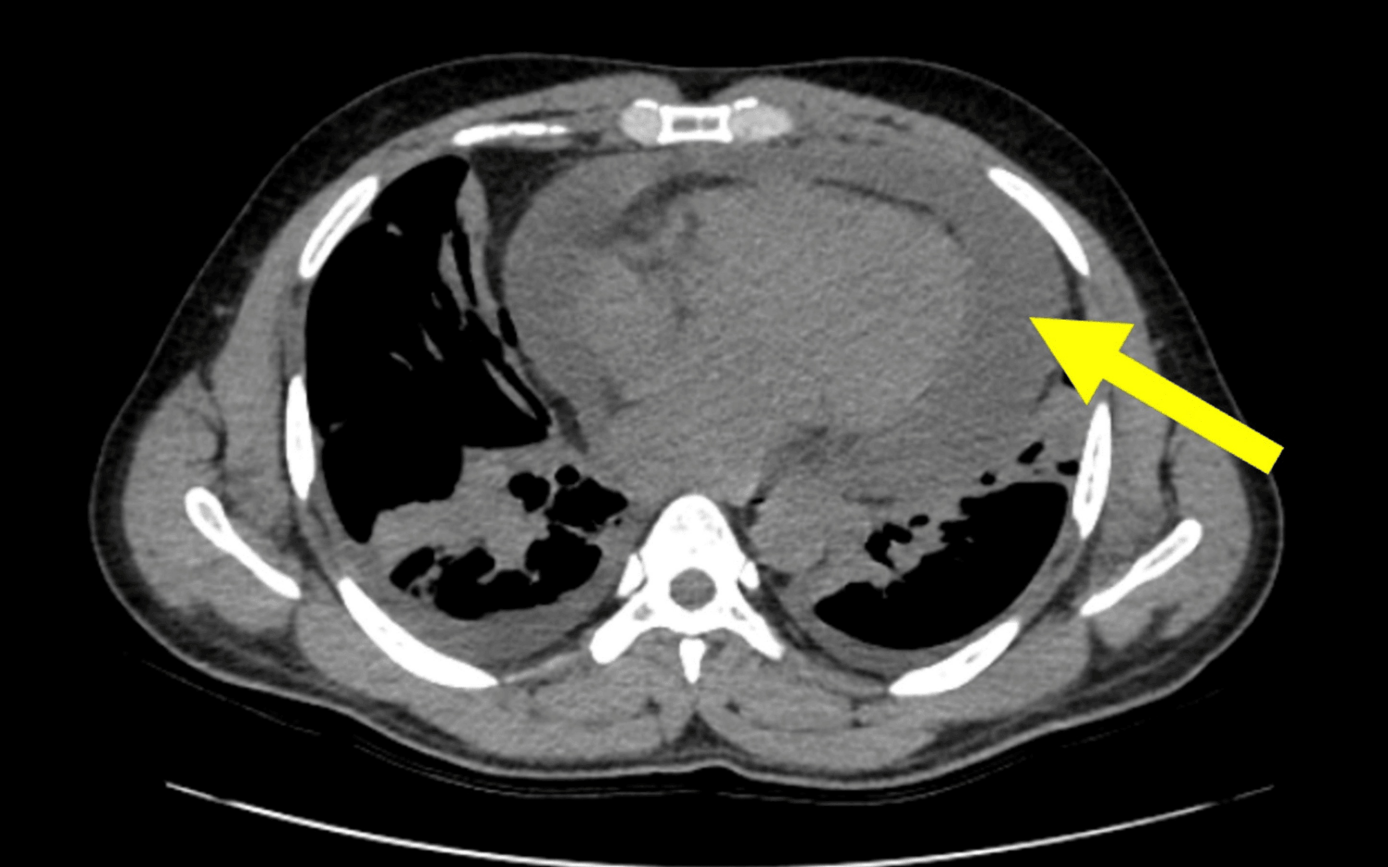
Computed tomography showed a large amount of pericardial effusion all around the heart.
